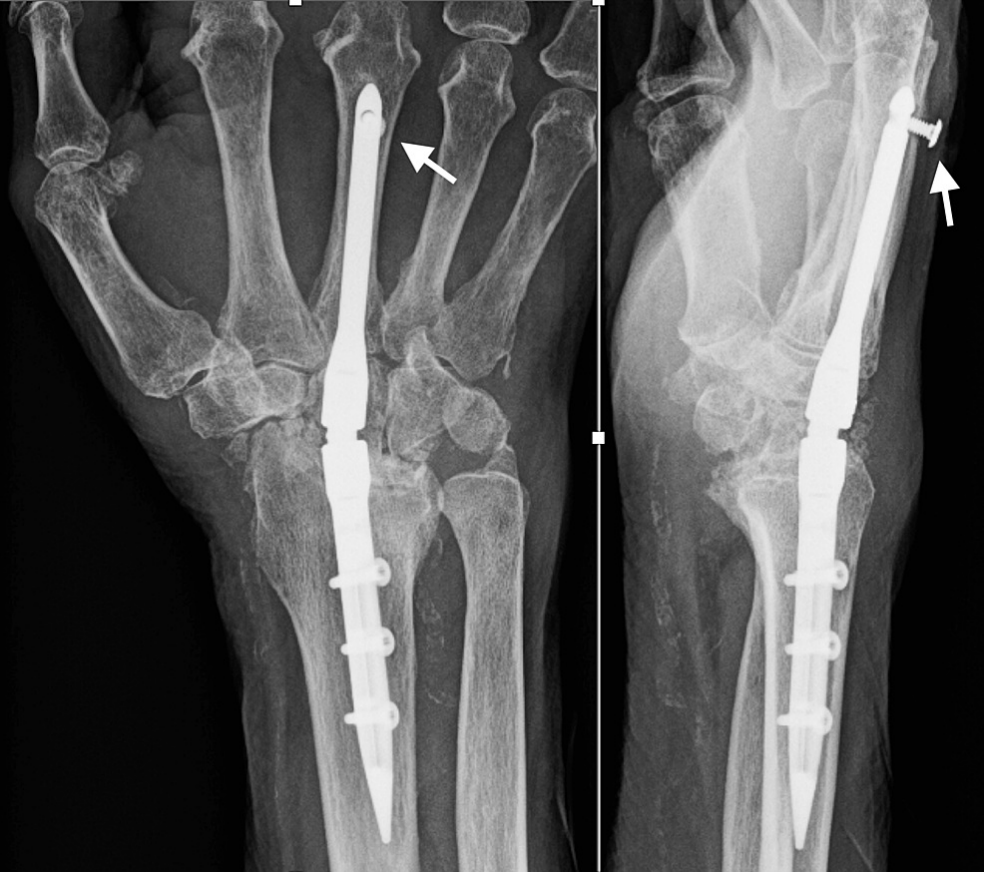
Follow-up radiographs of Case 2 Posteroanterior and lateral views at 1.5 months postop show that the distal unicortical screw backed out. The white arrows show the distal screw loosening and migration.
